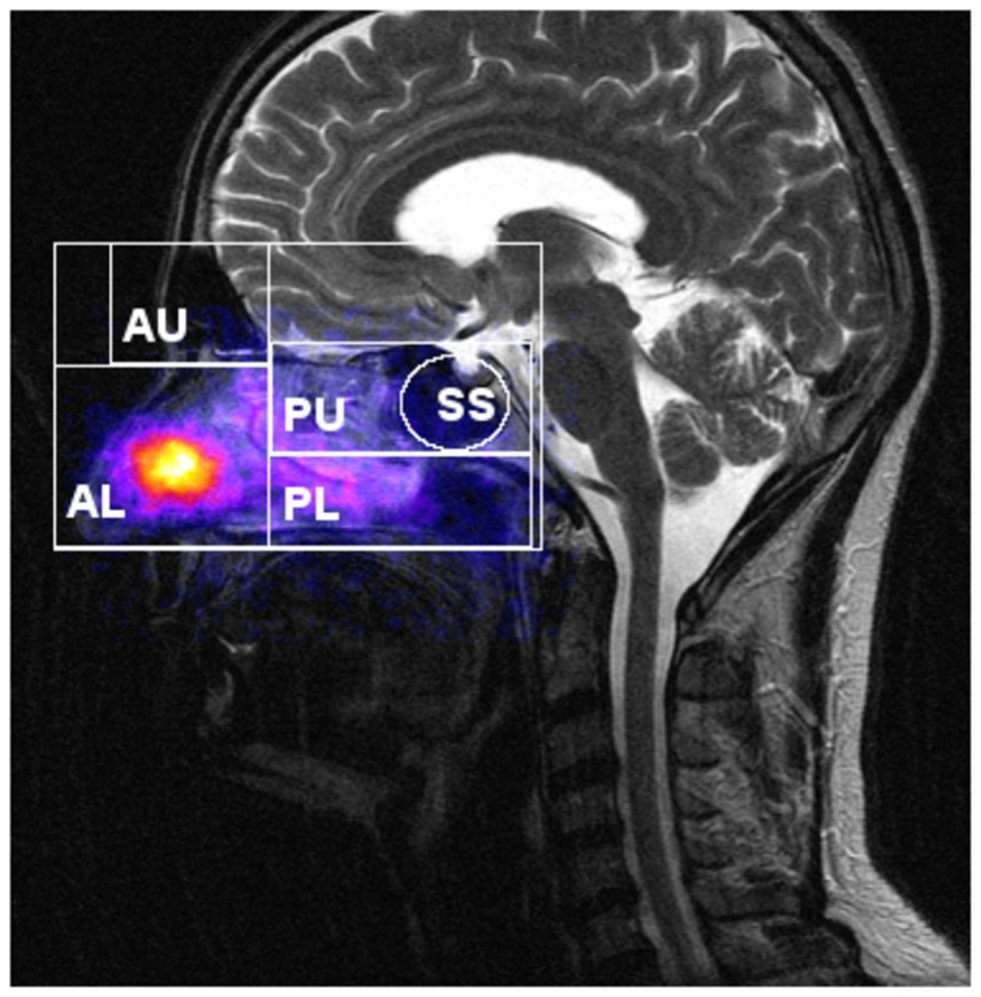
Definition of regions of interest (ROI’s) in lateral gamma camera images. Superposition of the lateral gamma camera image with a representative sagittal CT slice in a healthy volunteer and definition of the different ROI’s: total nasal (TN), anterior upper (AU: frontal sinuses), anterior lower (AL: nostrils, nasal valve and first cm of inferior turbinate), posterior lower (PL: turbinates, nasal floor, hard and soft palate), and posterior upper (PU: upper posterior nasal cavity, middle turbinate, ethmoidal and sphenoidal sinuses), and sphenoidal sinuses (SS).
